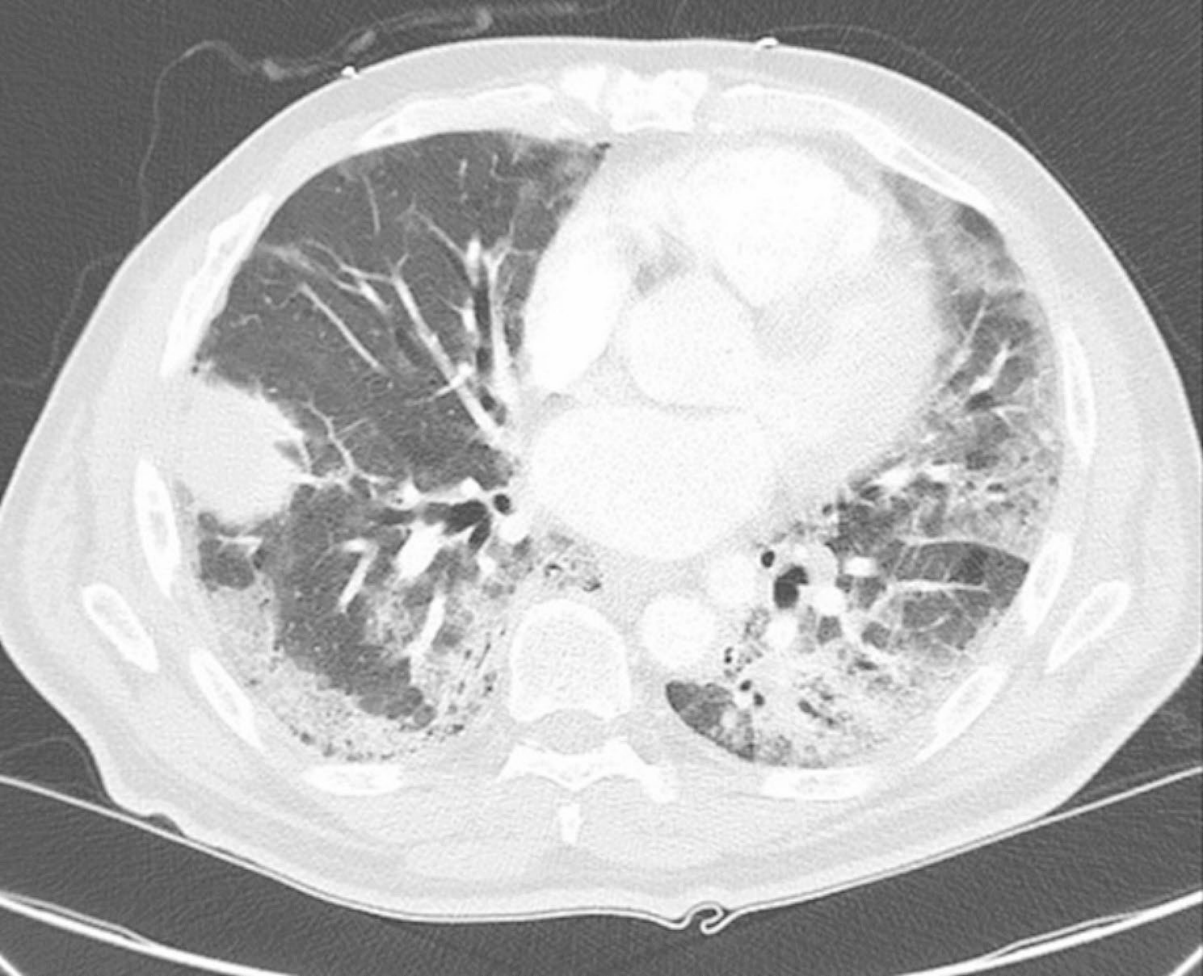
Computed tomography of the chest of index case obtained on day-2 of hospital admission: Multiple bilateral ground glass opacities and consolidations. The exam was negative for pulmonary embolus (not shown)
